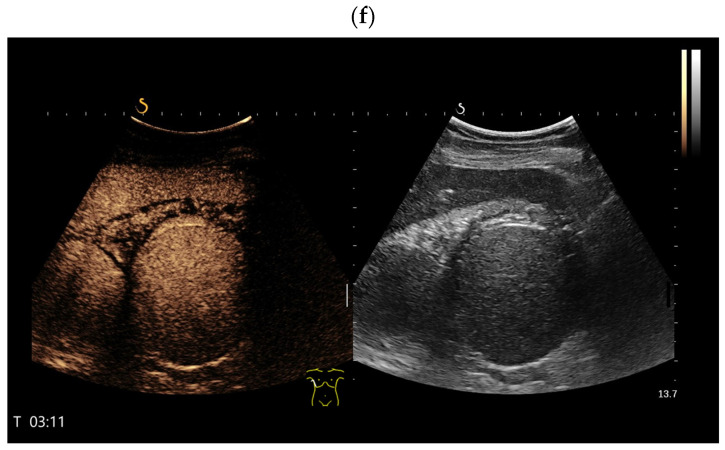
Kartagener syndrome (immobility of cilia in the airways and recurrent infections of the respiratory tract, situs inversus, bronchiectases, sinusitis). History of traumatic splenectomy following a motor vehicle accident. The transducer is positioned in the right 10th–11th intercostal space, demonstrating the liver extending far to the right due to situs inversus. Under normal anatomical conditions, the hepatic hilum would be visualized at this location. Distal to the transducer, two larger round structures (arrows) measuring up to 8 cm in diameter are identified (a). CDI and CEUS reveal a central feeding vessel with a branching vascular tree (b–d) as well as sustained contrast enhancement over several minutes (e,f), findings typical of accessory spleens. On B-mode imaging, several additional adjacent structures cannot be reliably delineated; however, contrast-enhanced ultrasound clearly demonstrates these lesions as further splenunculi. In the context of situs inversus and Kartagener syndrome, if splenic structures are located on the right side, and several large structures are detected, polysplenia should be considered as the differential diagnosis rather than splenosis.
